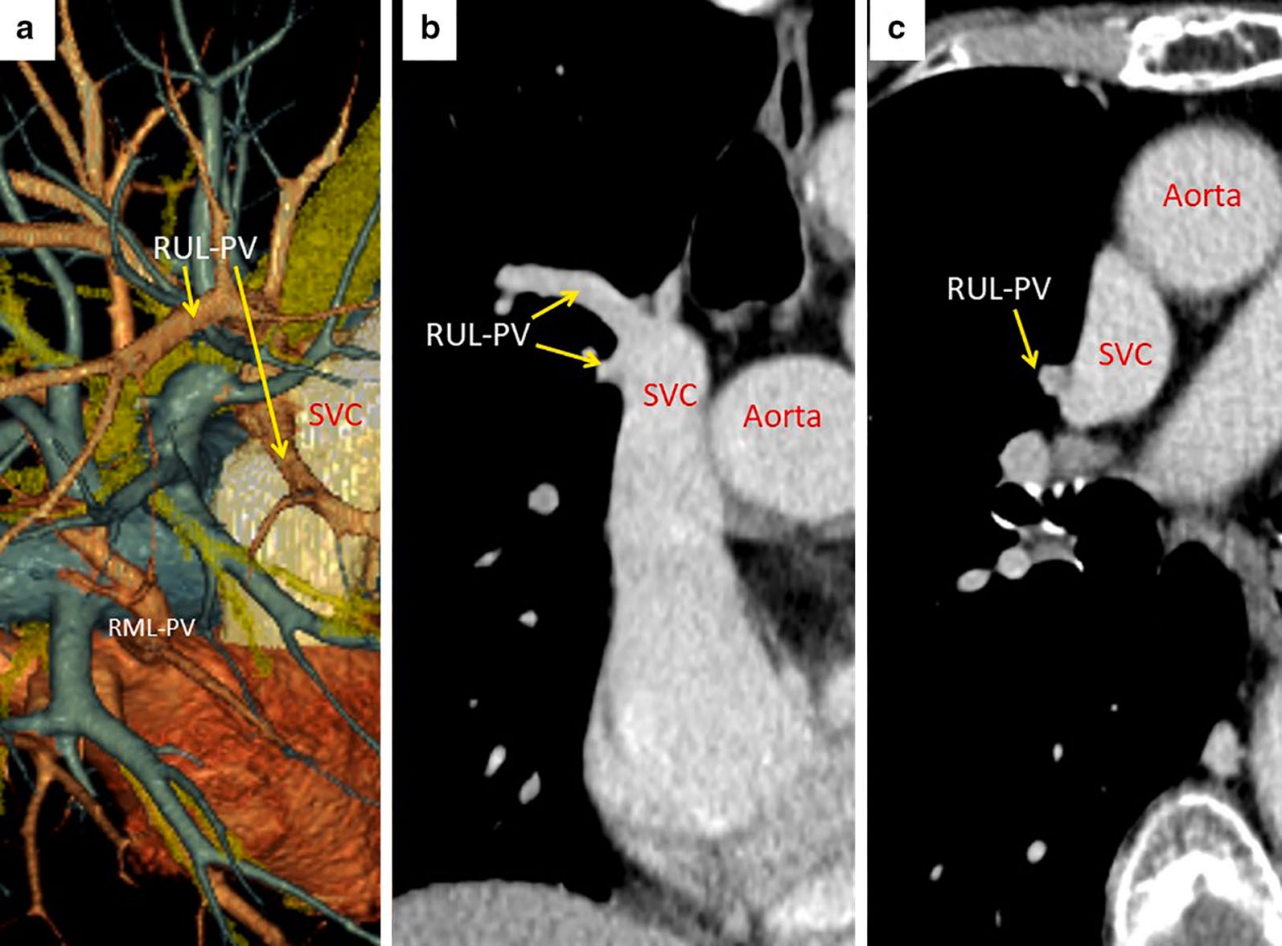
Three-dimensional computed tomography (CT) angiography (a) and enhanced CT (b: coronal view, c: axial view) revealed the partial anomalous pulmonary venous connection in the right-upper lobe returning into the superior vena cava (SVC). *RUL* right-upper lobe, *RML* right-middle lobe, *PV* pulmonary vein
